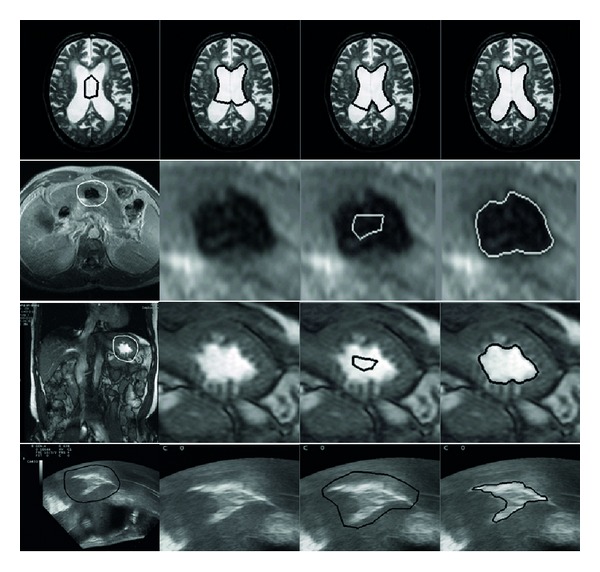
Experiments on MRI and ultrasound images.
